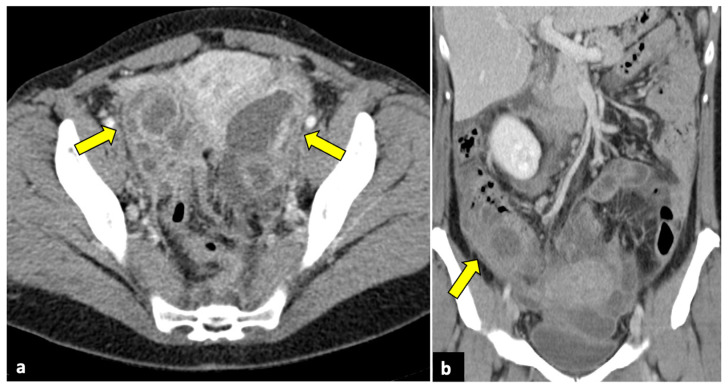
Bilateral tubo-ovarian abscesses in a 44-year-old woman who presented with fever, leucocytosis, and acute pelvic pain. CT axial (a) and coronal (b) pelvic scans demonstrate thick-walled, peripherally enhancing, multi-cystic, and tubular structures (yellow arrows), which proved to be bilateral tubo-ovarian abscesses at the time of surgery.
